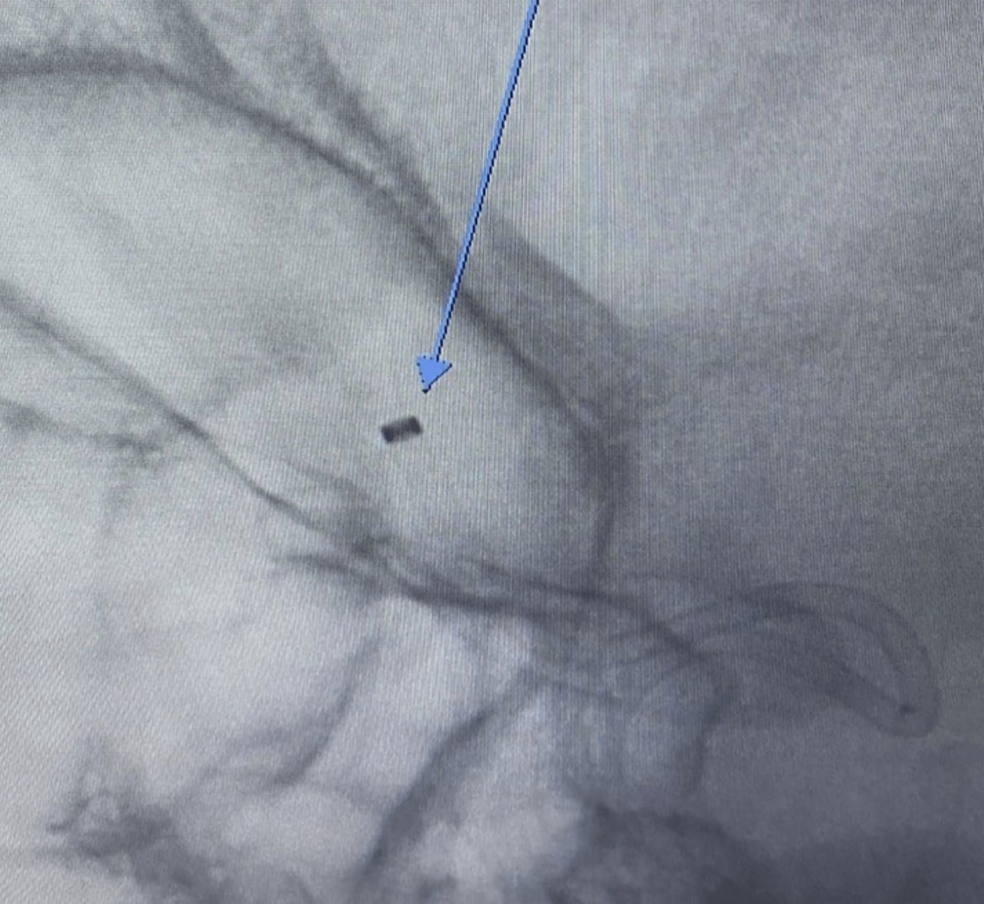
Oblique CT arteriogram showing the aspiration catheter in occluded M2
